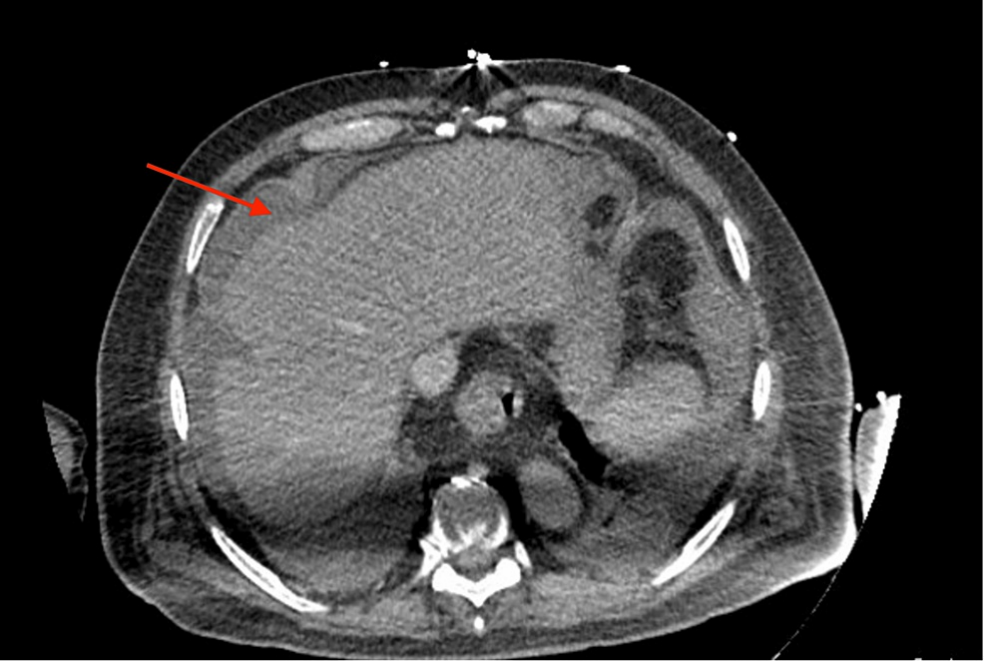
Computed tomography scan of sub-capsular hematoma
